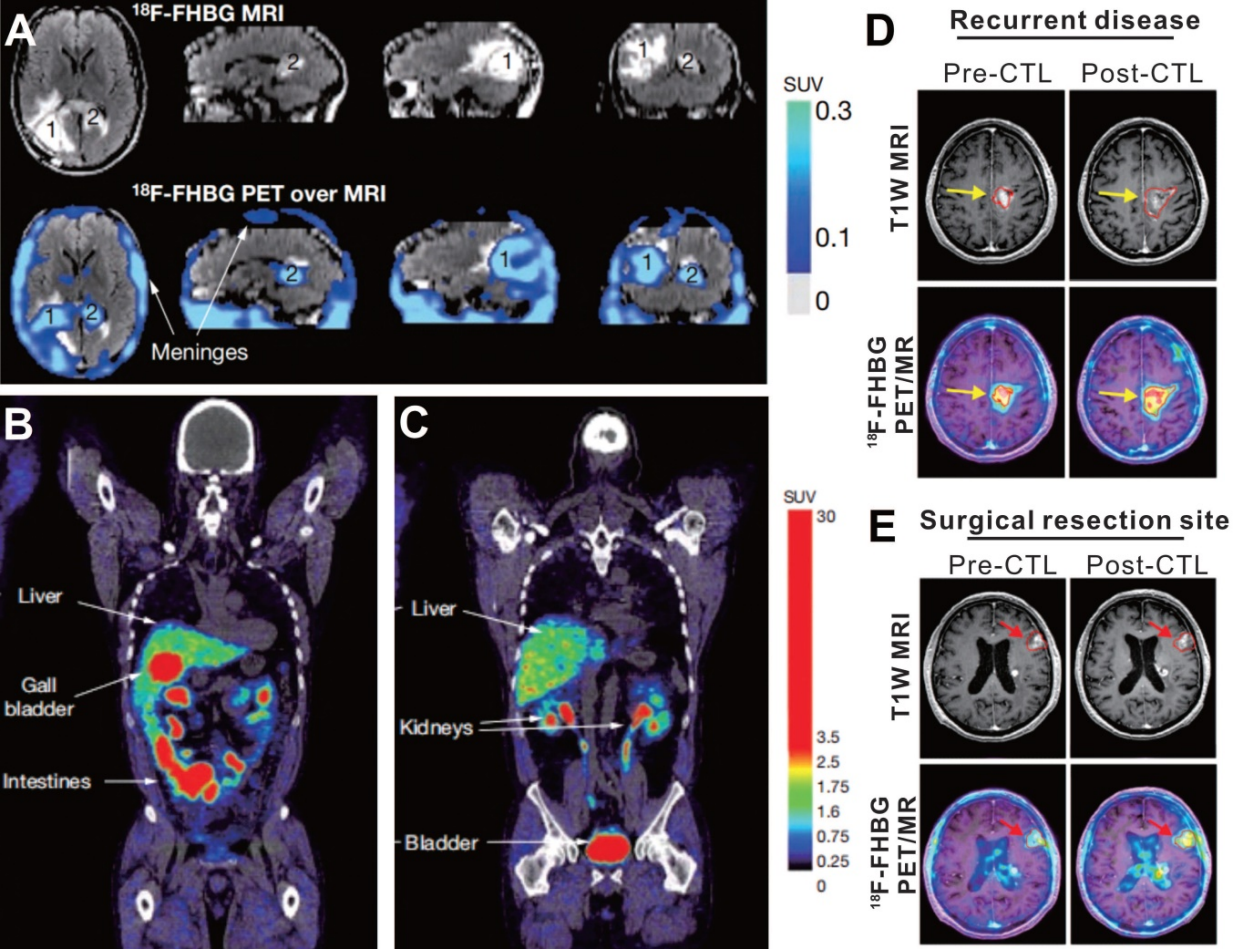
^18^F-FHBG PET imaging based on HSV-1 tk reporter enables CAR-T cell visualization in patients with glioblastoma. A. Images of brain MRI and ^18^F-FHBG PET/MRI superposition in a patient who received IL-13- and HSV-1 tk- expressing T cells. Both the surgically resected tumor site (lesion 1, which received CAR-T injection) and the unresected tumor (lesion 2, which did not receive CAR-T injection) had higher activity in contrast to the normal brain background. B-C. Sites of physiologic uptake of ^18^F-FHBG PET/CT included the liver, gallbladder, intestines, kidneys, and bladder. D-E. Pre- and Post-CTLs ^18^F-FHBG PET/MR images in recurrent disease (D, received CAR-T injection) and at untreated tumor (E) sites in a 60-year-old man with multifocal left hemispheric glioma. ^18^F-FHBG PET images showed the recurrent tumor and untreated tumor (B2, red arrows) had obvious activity that increased after CTLs treatment. A-C adapted with permission from 64, copyright 2008 Springer Nature, and D-E from 77, copyright 2017 American Association for the Advancement of Science.
